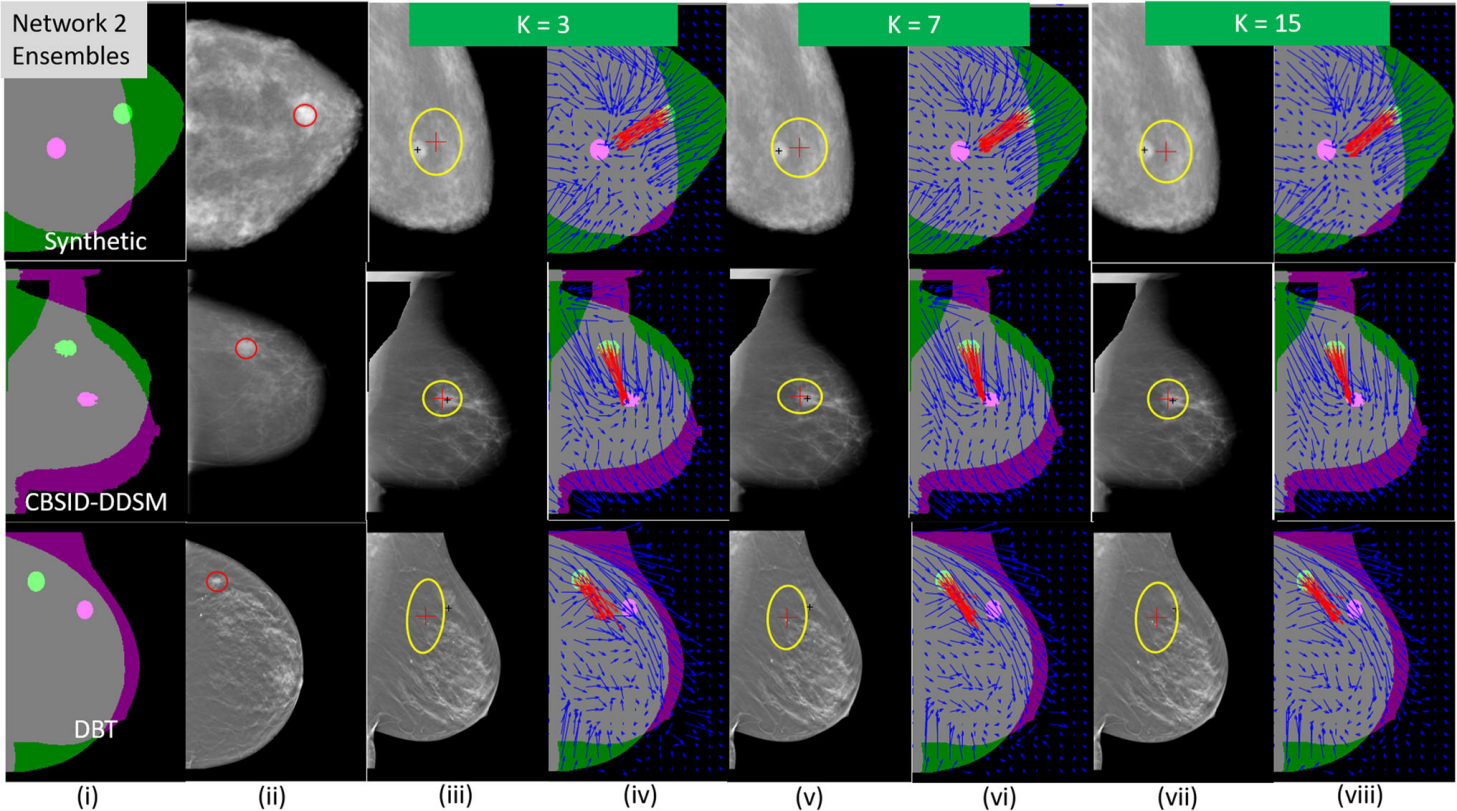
Example uncertainty ellipses with corresponding deformation fields shown for synthetic (top row), digitized scanned film [CBIS-DDSM] (middle row), and slices from DBT (bottom row) X-ray data. Color schemes in cols. i, iii, v, and vii are the same as Fig. 8. In col. ii, the red circle represents the CC lesion location. In cols. iv, vi, and viii, the deformation vectors, displayed as quiver plots, show the individual pixel displacements. The red vectors represent pixels displaced from the CC lesion position, and the blue vectors emanate from pixels at other locations across the breast
